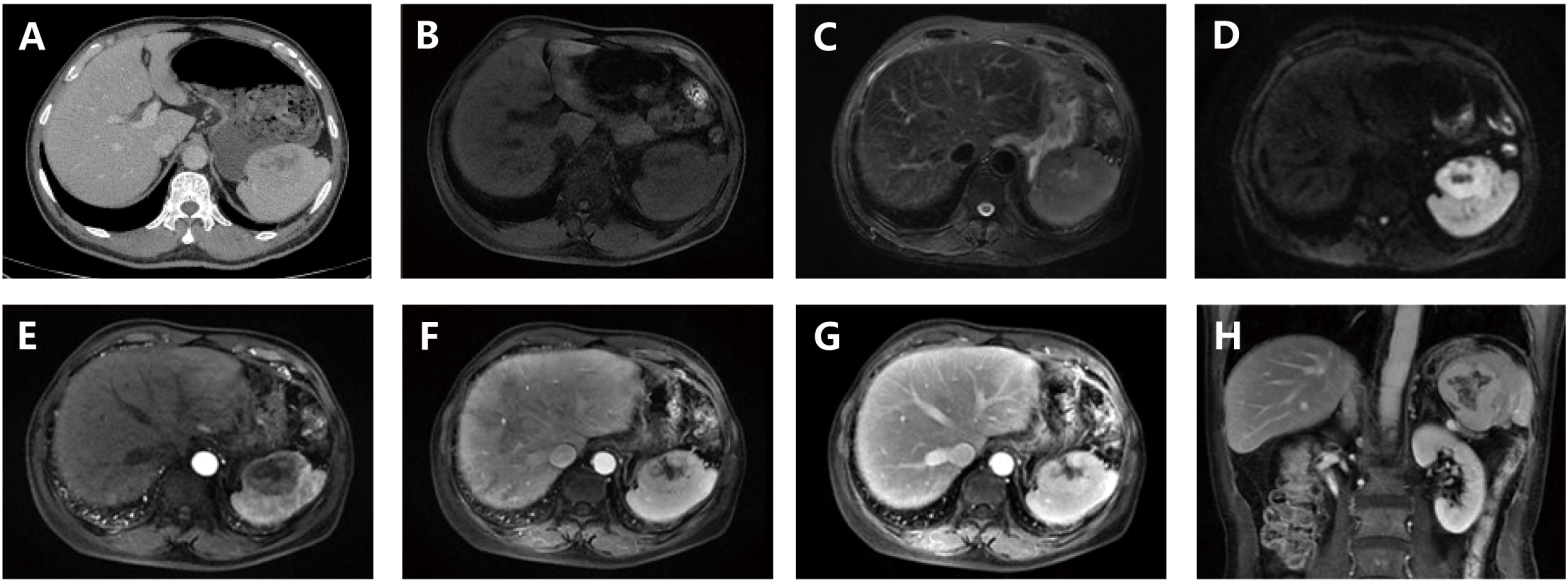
(A) Contrast-enhanced CT demonstrates a splenic space-occupying lesion (mass) exhibiting a rounded morphology with ill-defined margins. The solid components display mild contrast enhancement, while cystic necrosis and poorly-defined hemorrhage are observed. Absence of a capsule, calcification, or metastatic features is noted; (B) Pre-contrast MRI shows the lesion with peripheral isointensity and central hypointensity; (C) T2-weighted MRI demonstrates peripheral isointensity with central intermediate-to-hyperintense signal; (D) DWI) reveals peripheral hyperintensity surrounding a central hypointense region; (E–G) (axial): Post-contrast axial imaging reveals mild-to-moderate heterogeneous enhancement without evidence of encapsulation or fatty components; (H) (coronal): Post-contrast coronal imaging confirms mild-to-moderate heterogeneous enhancement without evidence of encapsulation or fatty components.
